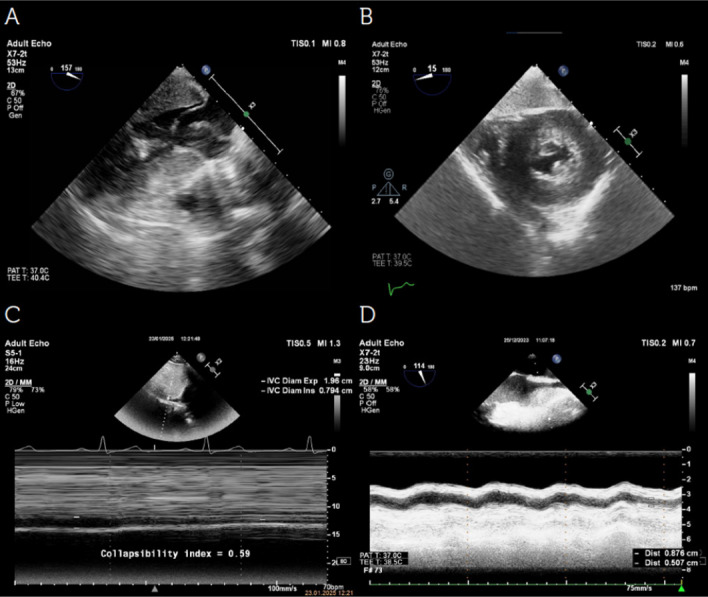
Echocardiographic assessment of volume status. A, B) Echocardiographic images demonstrating “kissing ventricles,” a hallmark of hypovolemia. In severe hypovolemia, left ventricular walls nearly touch at end-systole due to reduced preload, with minimal residual cavity. Additionally, diastolic filling is significantly impaired, further supporting volume depletion. C) Inferior vena cava (IVC) collapsibility index (IVC-CI) measurement using M-mode echocardiography in a spontaneously breathing patient. IVC-CI=(IVCmax–IVCmin)/(IVCmax)x100. >50% suggests a low central venous pressure (< 5 mmHg), indicating hypovolemia. IVC distensibility index (IVC-DI) is applied in mechanically ventilated patients and is calculated as follows: IVC-DI=(IVCmax–IVCmin)/(IVCmin)x100. >18% suggests fluid responsiveness. D) Superior vena cava (SVC) collapsibility index (SVC-CI) measurement using transesophageal echocardiography, which is primarily applied in mechanically ventilated patients. SVC-CI=(SVCmax-SVCmin)/(SVCmax)x100. >36-40% suggests volume responsiveness.
